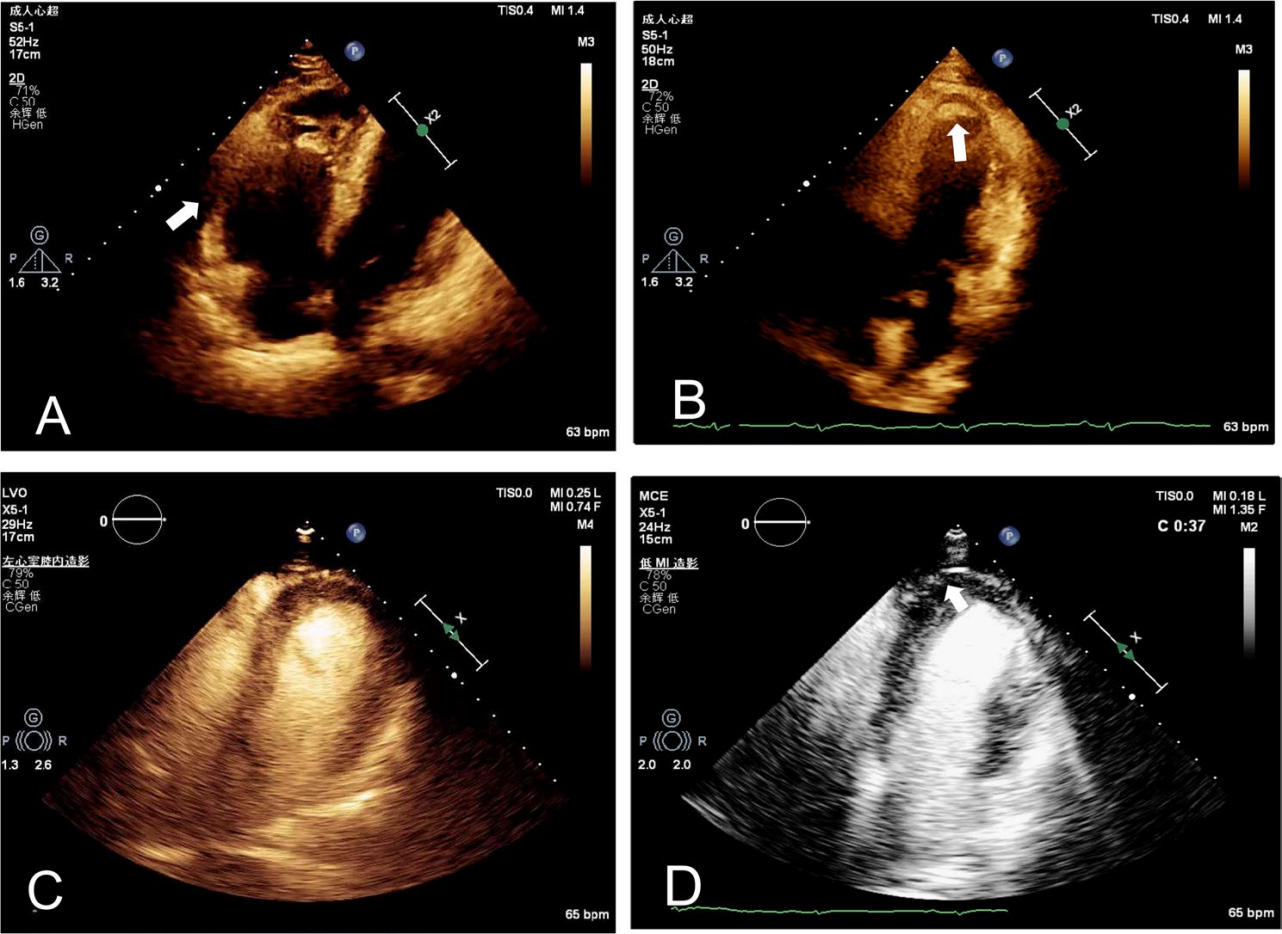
A, Echocardiography showed right ventricular enlargement with thinning of the ventricular walls and reduced motion of the right ventricular wall; B, Echocardiography showed a flake isoechoic lesion in the apex of the LV; C, LVO reveals no apparent filling defect at the apex and reduced motion in the anterior, lateral, inferior segments of the left ventricular apex; D, MCE patten shows markedly lower perfusion levels of the subepicardial myocardium at the left ventricular apex compared to the endocardial enhancement at the same level
